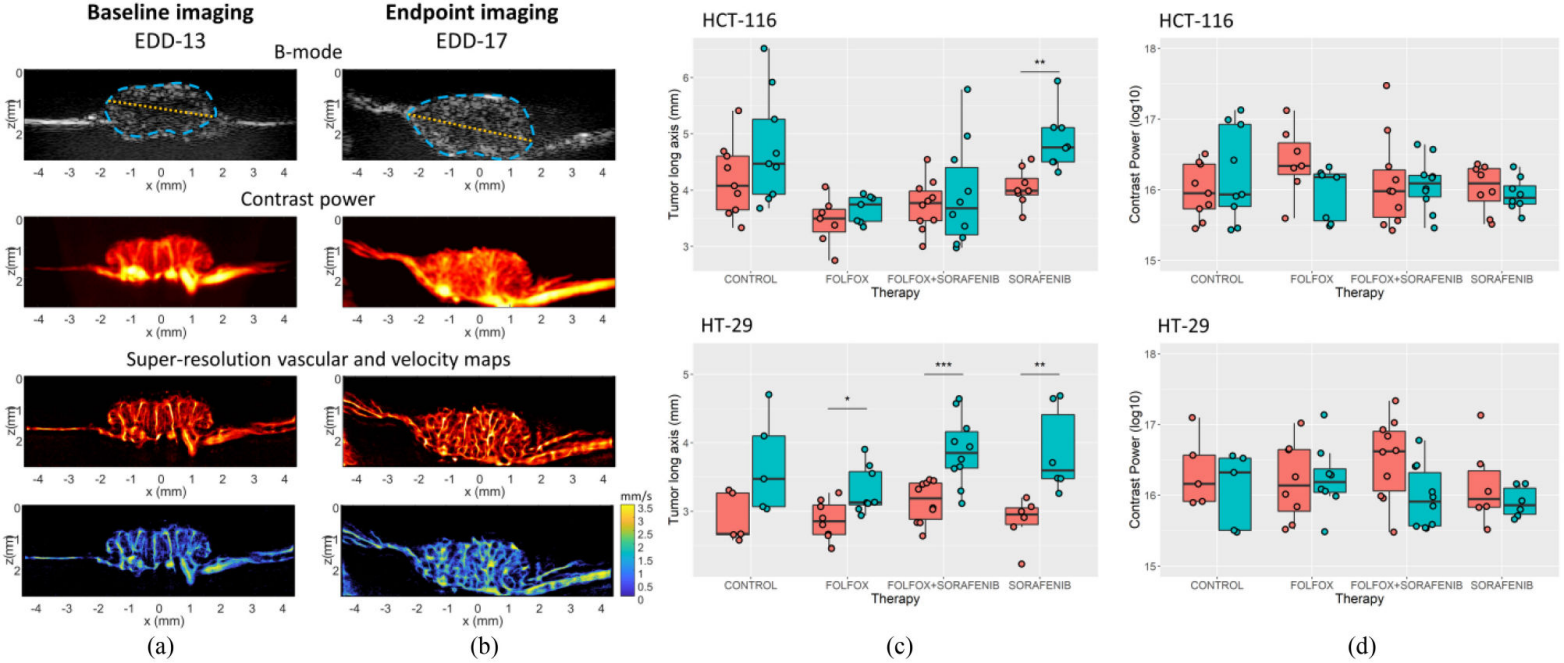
Longitudinal changes in CAM tumors. A) Representative baseline contrast-enhanced power Doppler and super-resolution ULM images of CAM engrafted tumors. B) This same control tumor at endpoint imaging, which demonstrates both the growth of the tumor diameter and the continued development of intratumor vascularization over the course of the study. Tumor outlines and maximal diameter are demonstrated as cyan and orange lines on the B-mode image, respectively. C) Quantification of the tumor long-axis which reveals a general trend of tumor growth for all treatment groups in this study. D) Quantification of the contrast power of the tumor cross-sectional area.
